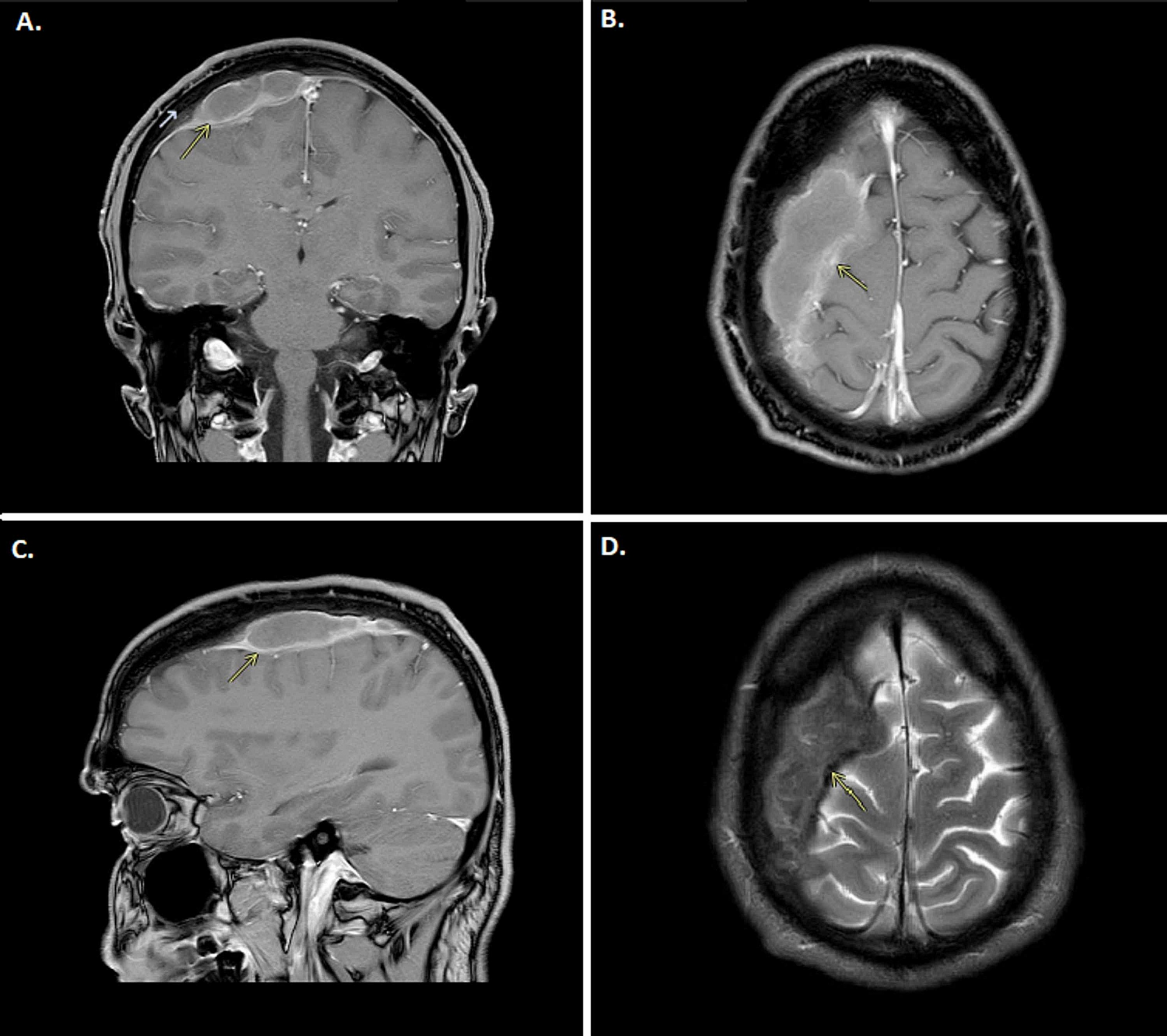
MRI scan of the brain. ABC; T1-weighted image with contrast, coronal, axial and sagittal view showing a mass with high signal intensity and peripheral contrast enhancement. D; T2-weighted image, axial plane that shows a low signal intensity of the collection.
